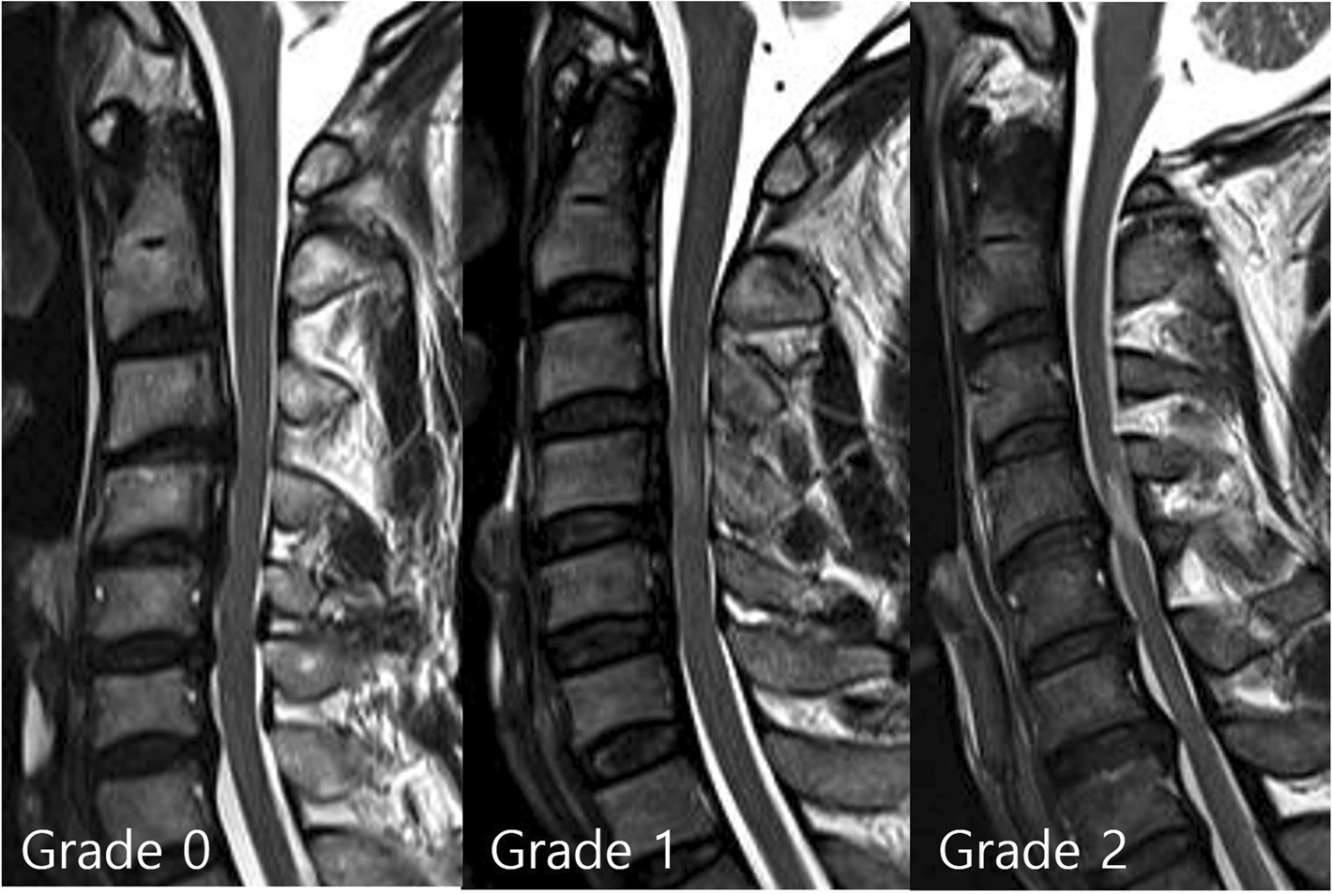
Signal intensity grade. Signal intensity of the spinal cord was assessed as grade 0—no change in signal intensity (SI) on T2-weighted MR images; grade 1—light intensity change; and grade 2—bright signal, clearly distinguishable from that of grade 1
